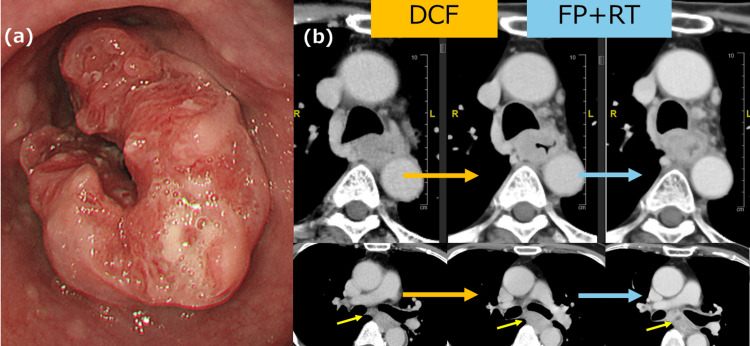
EGD and CT findings (a): Type 2 esophageal SCC, occupying two-thirds of the circumference of the upper middle esophagus. (b): One course of chemotherapy, DCF, slightly increased the size of the tumor; however, chemoradiotherapy (FP+RT) decreased the size of the tumor. The lymph nodes in the tracheal bifurcation demonstrated enlargement. Post-chemoradiotherapy imaging revealed a low-density region within the lymph nodes, consistent with necrosis (yellow arrow). EGD: esophagogastroduodenoscopy; CT: computed tomography; SCC: squamous cell carcinoma; DCF: docetaxel, 5-fluorouracil, and cisplatin; FR: 5-fluorouracil and cisplatin
